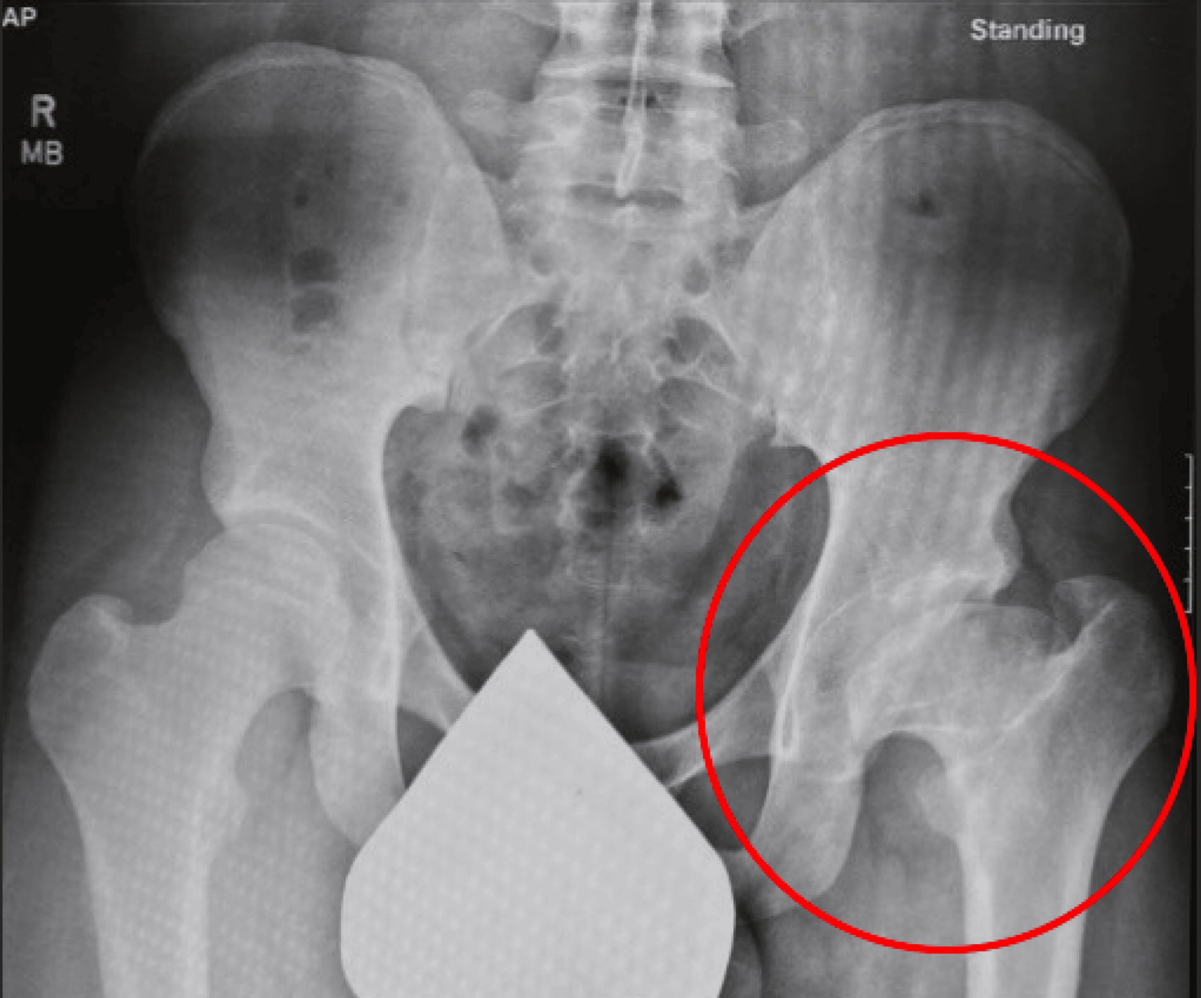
Standing anteroposterior pelvic X-ray taken on December 4, 2019.
